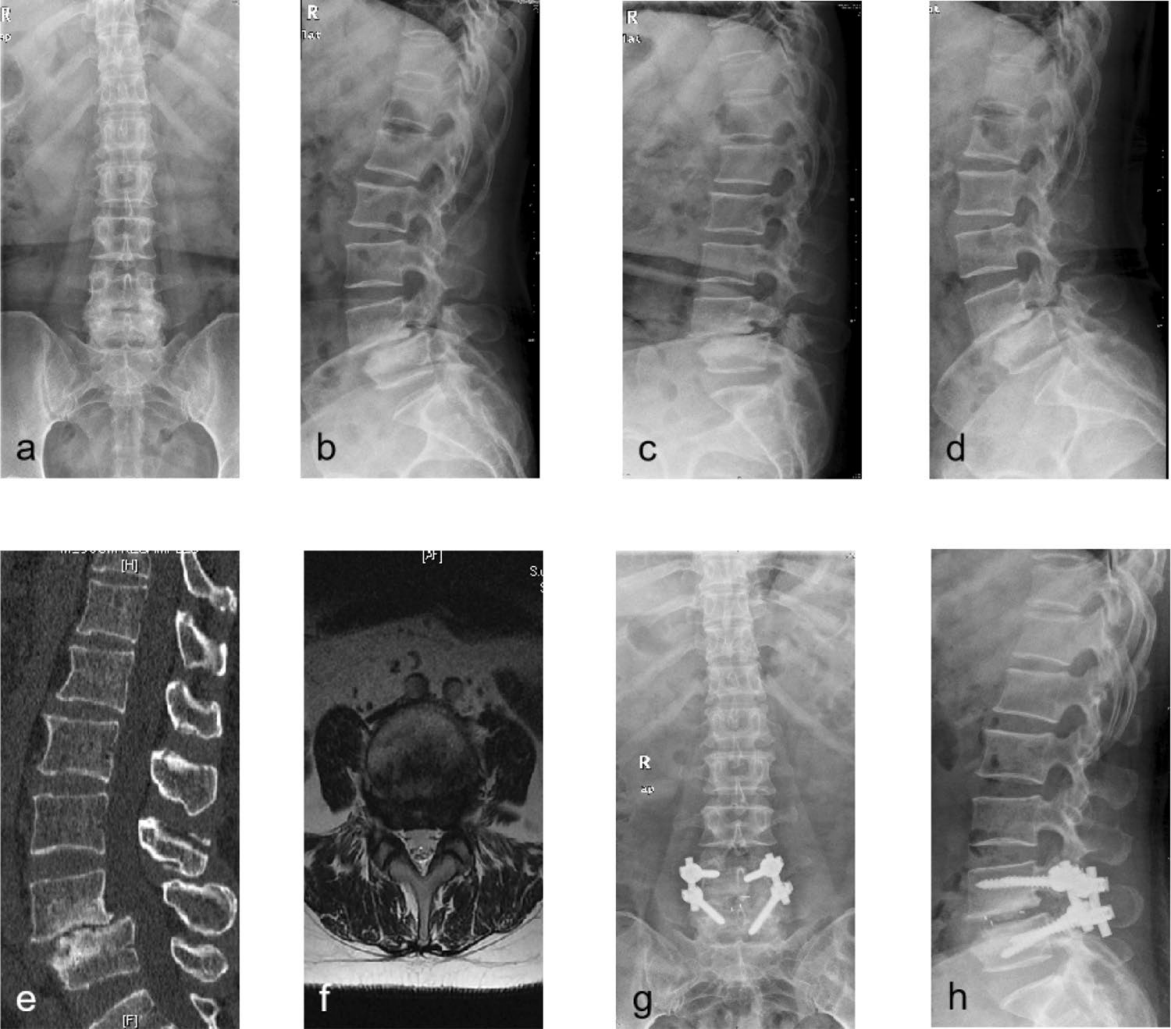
A 57 years old female patient who suffered from low-back pain with radicular pain of left lower extremity for 7 months prior to admission underwent TO-TLIF procedure. (a,b) Preoperative antero-posterior and lateral plain radiography; (c,d) preoperative flexion and extension plain radiography indicated patient with LDS grade 1; (e,f) preoperative sagittal CT scan and axial T2-weighted MR image; (g,h) antero-posterior and lateral plain radiography after surgery.
